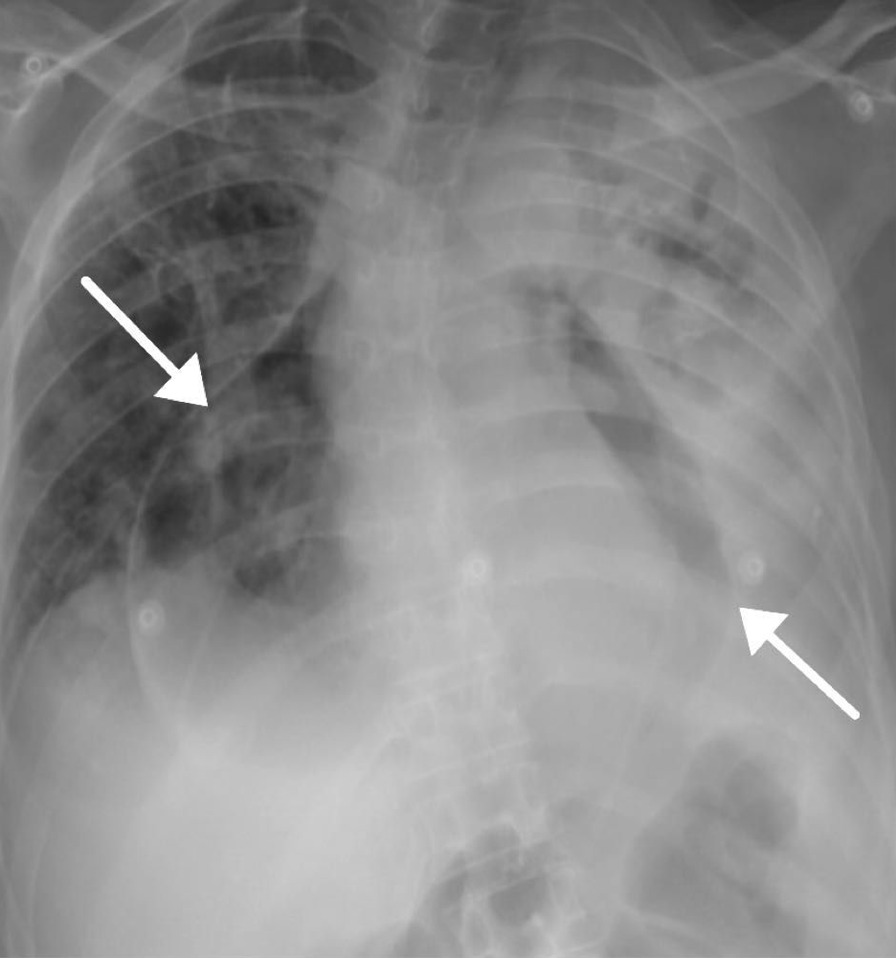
Chest radiographs revealing severe hydro-pneumopericardium, total opacification of the left lung, and air space consolidation. The arrows refer to hydro-pneumopericardium, total opacification of the left lung, and air space consolidation
